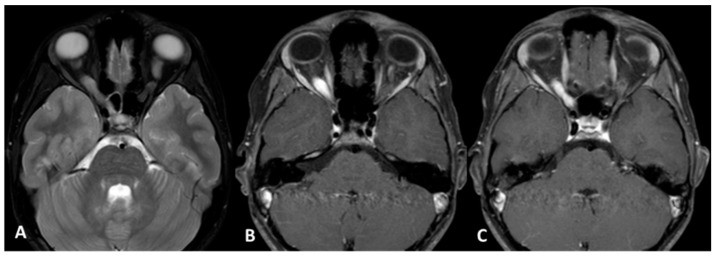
Optic glioma 1Aright (Subject 12 OPG). Orbit MRI. (A) Axial T2-weighted images (w.i.) with fat suppression; (B,C) Axial T1 w.i. with fat suppression and after gadolinium administration show enlargement of the optic nerve in the orbit and in the optic canal on the right side with intense and homogenous enhancement. OPG: Optical pathways glioma; MRI: Magnetic resonance imaging.
